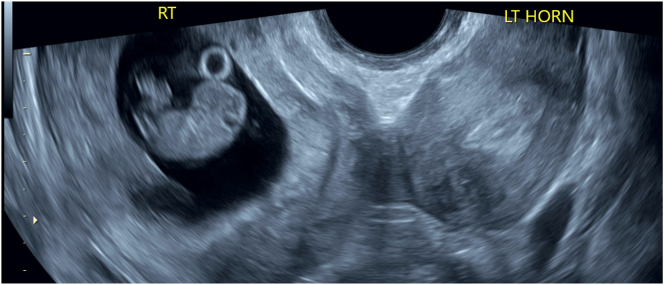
Dating ultrasound at 8 weeks gestational age showing a fetus in the right uterine horn and an empty left uterine horn.
